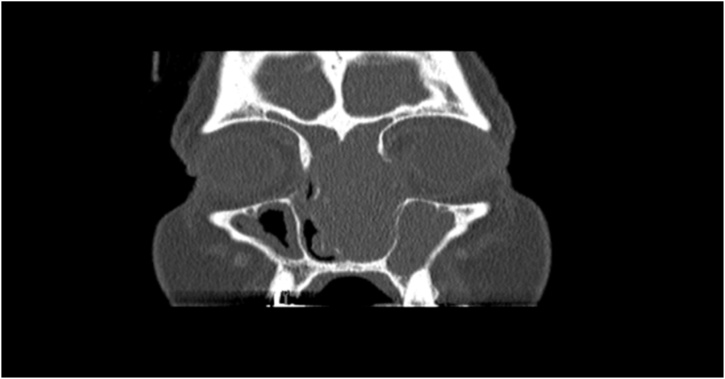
Preoperative CT coronal Cut showed left nasal mass with bony invasion.
